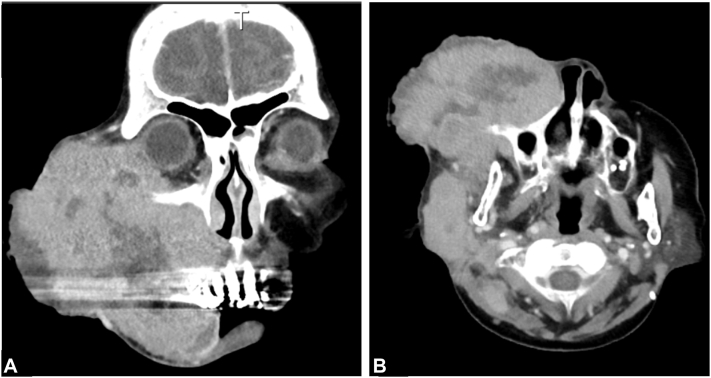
Computed tomography of the primary Merkel cell carcinoma lesion (right cheek) at the initial examination. A, Coronal view. B, Axial view.
